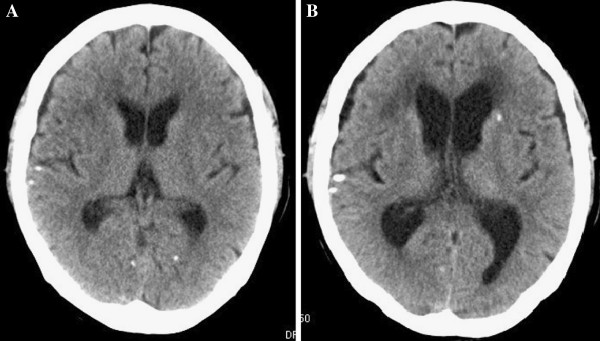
Brain computed tomography imaging without intravenous contrast (A) and (B) showed tiny calcified lesions in both cerebral hemispheres (A and B) and enlarged lateral ventricles (B).
